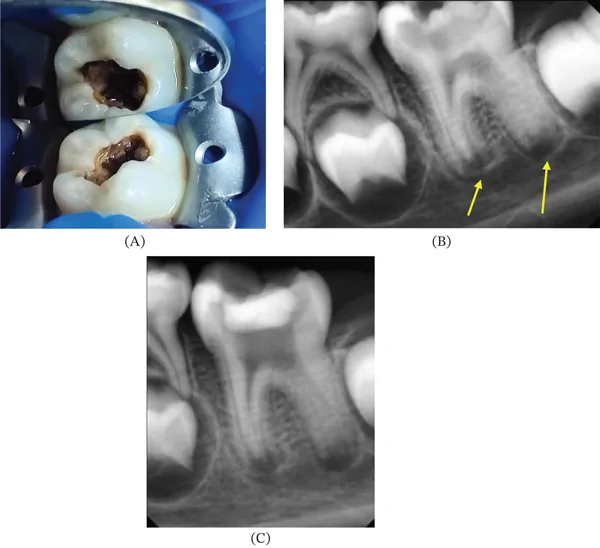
(A) Preoperative intraoral clinical photograph showing tooth 36 (deeply carious) under rubber dam isolation. (B) Diagnostic intraoral periapical (IOPA) radiograph showing tooth 36 (open apices visible). (C) IOPA radiograph after triple antibiotic paste placement into the canals and coronal seal with Cavit.
